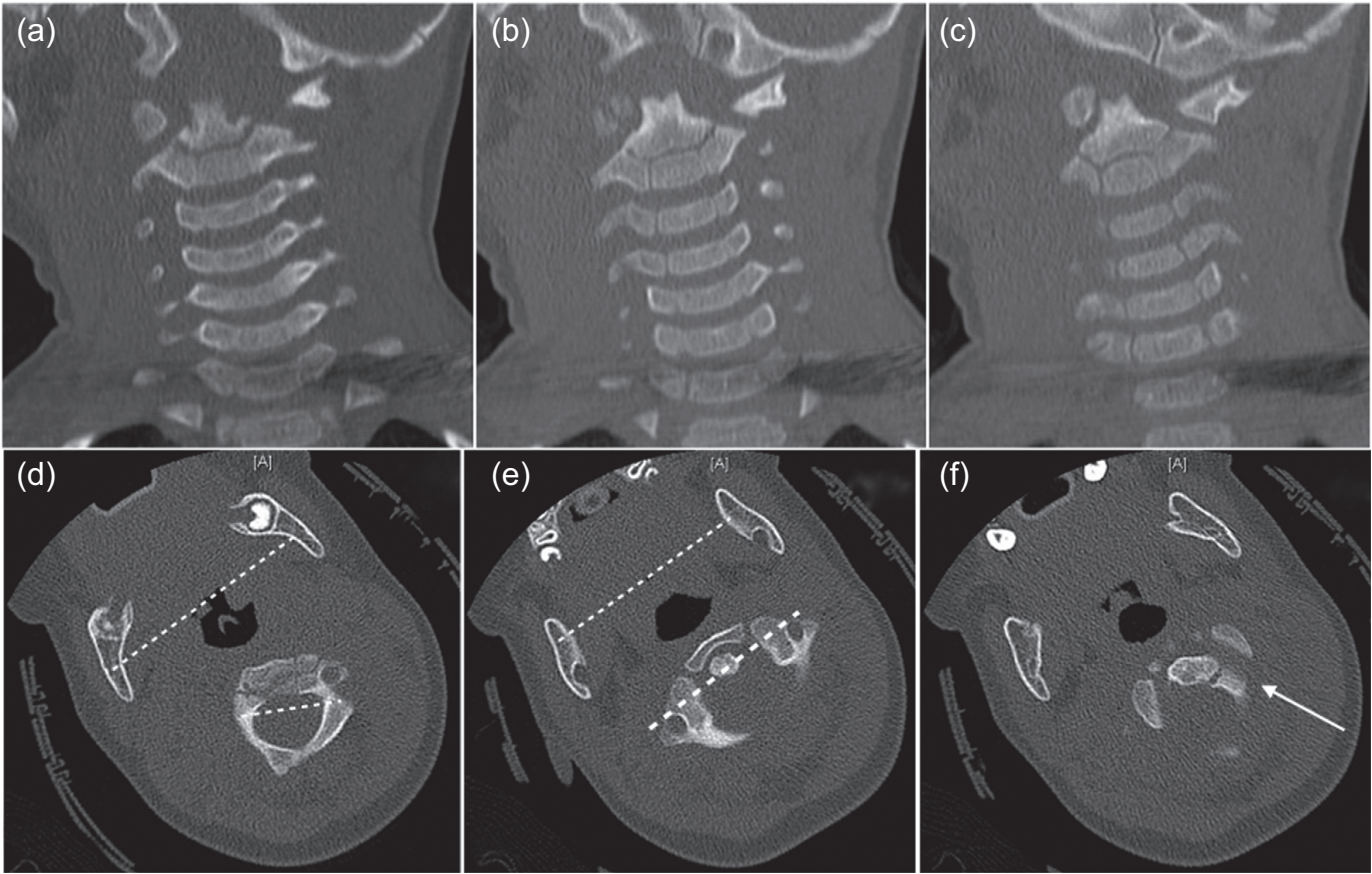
Case 1: A 16-month-old boy who sustained a motor vehicle accident. (a–c) Pre-reduction computed tomography (CT) scans showing the asymmetrical distance between dens and C1 lateral mass on both sides. (d) The difference between the transverse axis of the skull and C2. (e) The alignment of the transverse axis of C1 and skull. (f) The dislocated C1/2 joint on the left side (arrow).
